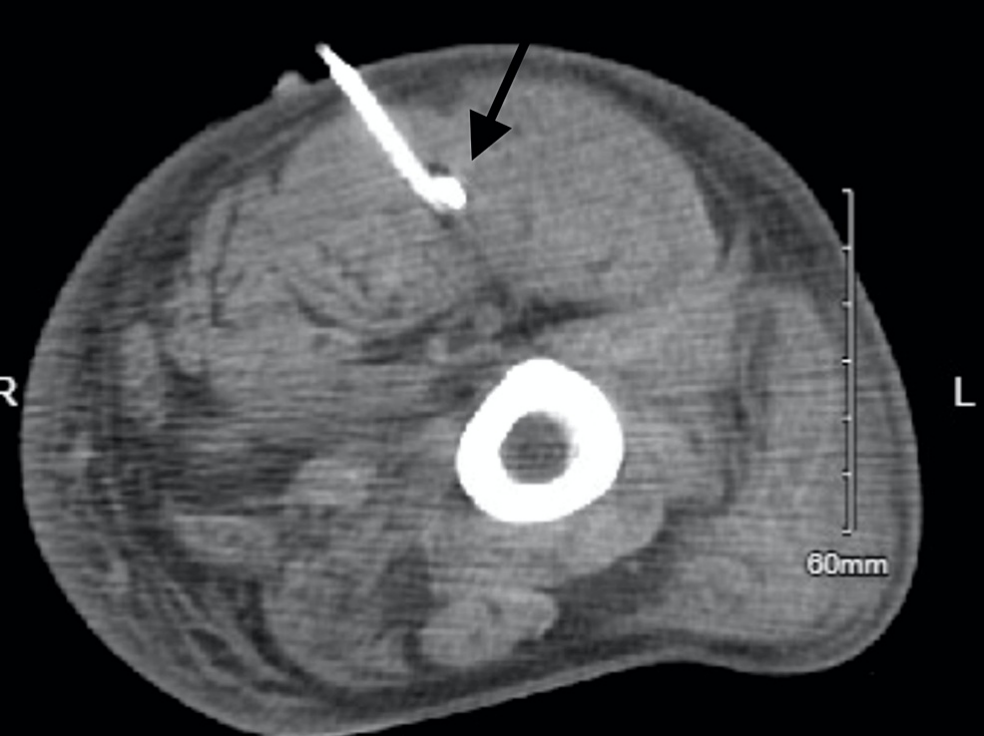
CT scan with axial view showing percutaneous drainage of a 20cm x 4.5cm x 4cm posterior thigh fluid accumulation
